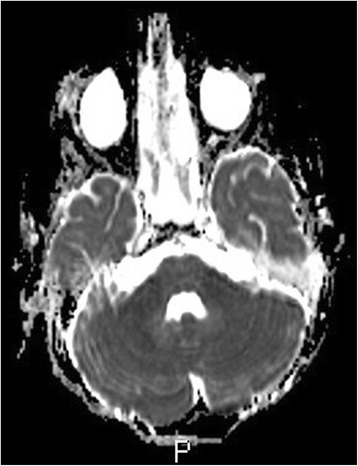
MRI brain after administration of mdc nuanced impregnation of the meninges in the cerebellar lobe in some places shows micro nodular appearance (case 4)
